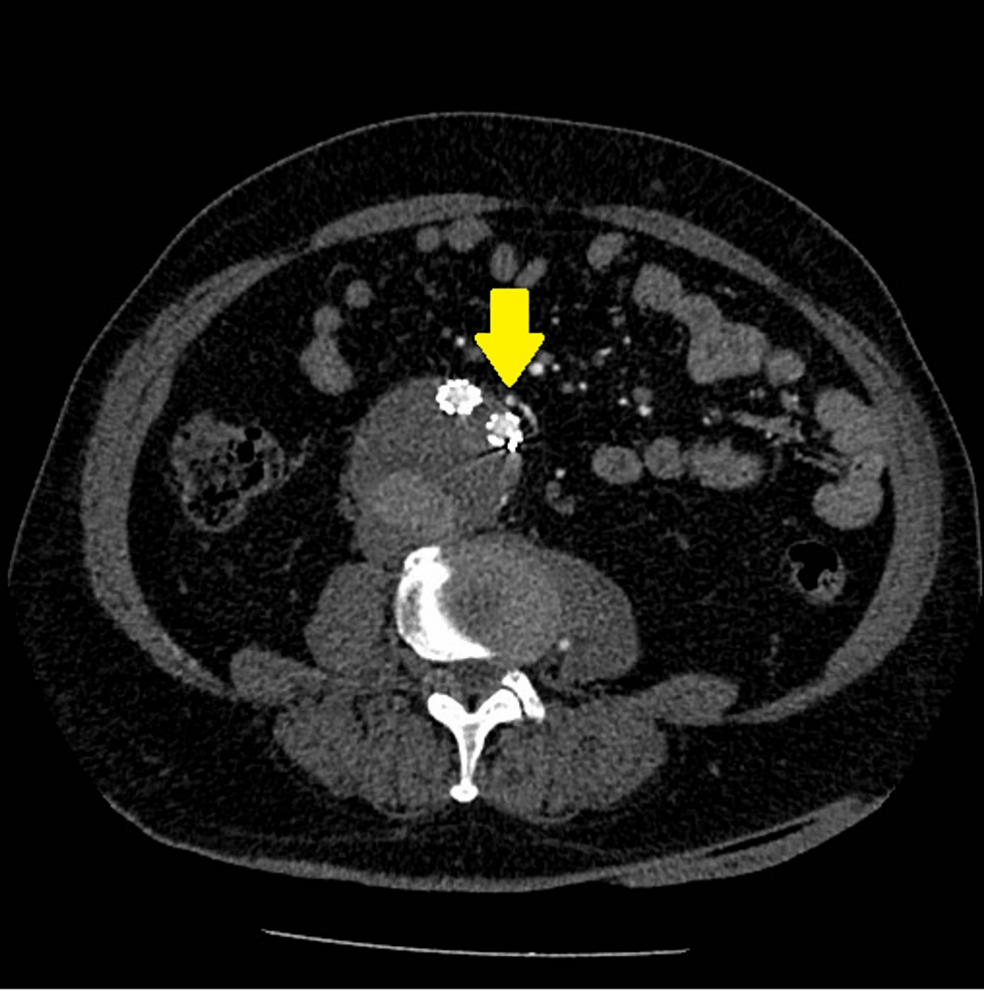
Pre-operative computed tomography angiography shows patent inferior mesenteric artery leading to endoleak type II.
